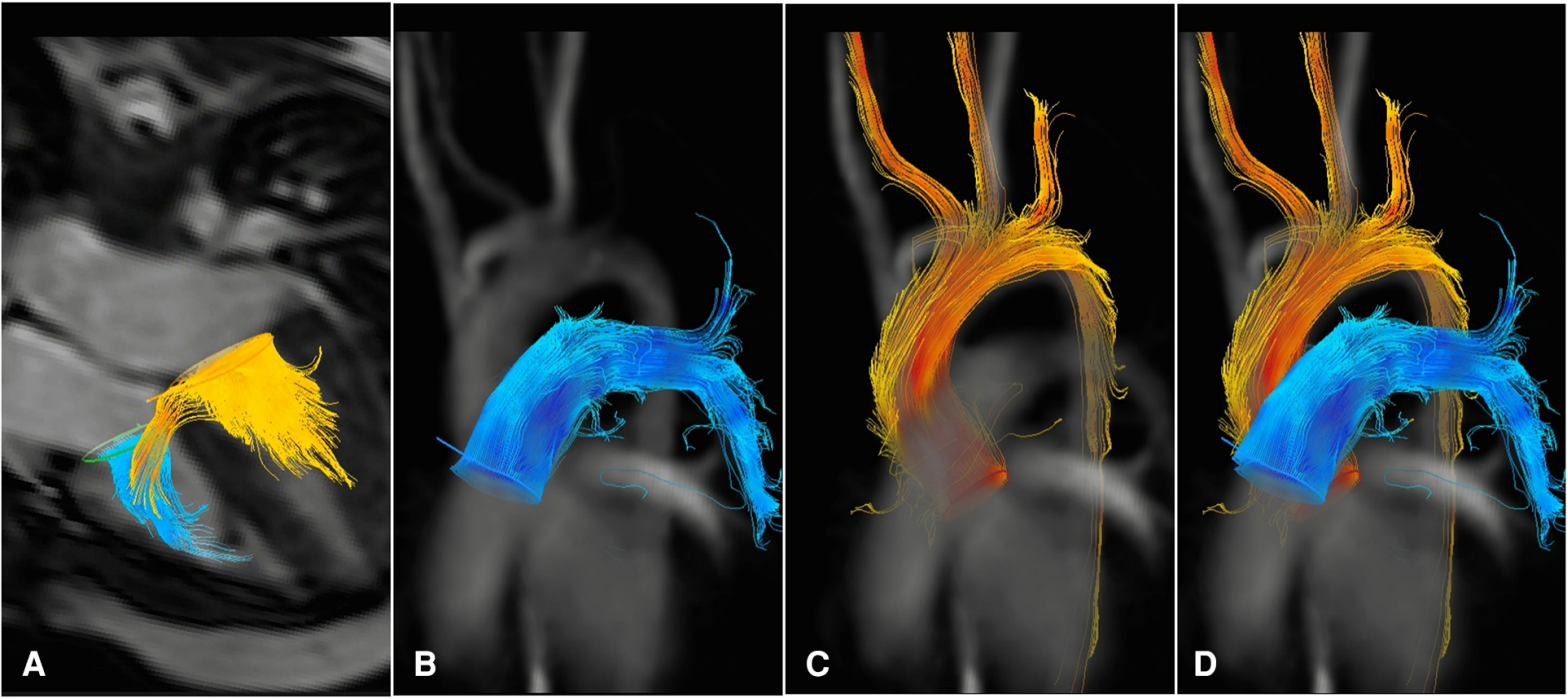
4d flow MRI images from a patient with VSD. The blood flow connection between the left and right ventricles can be visualized as yellow streamlines traversing through the ventricular septum during diastole (A) Blood flow measurements during systole are shown in the main pulmonary artery (B–D) and the ascending aorta (C–D).
